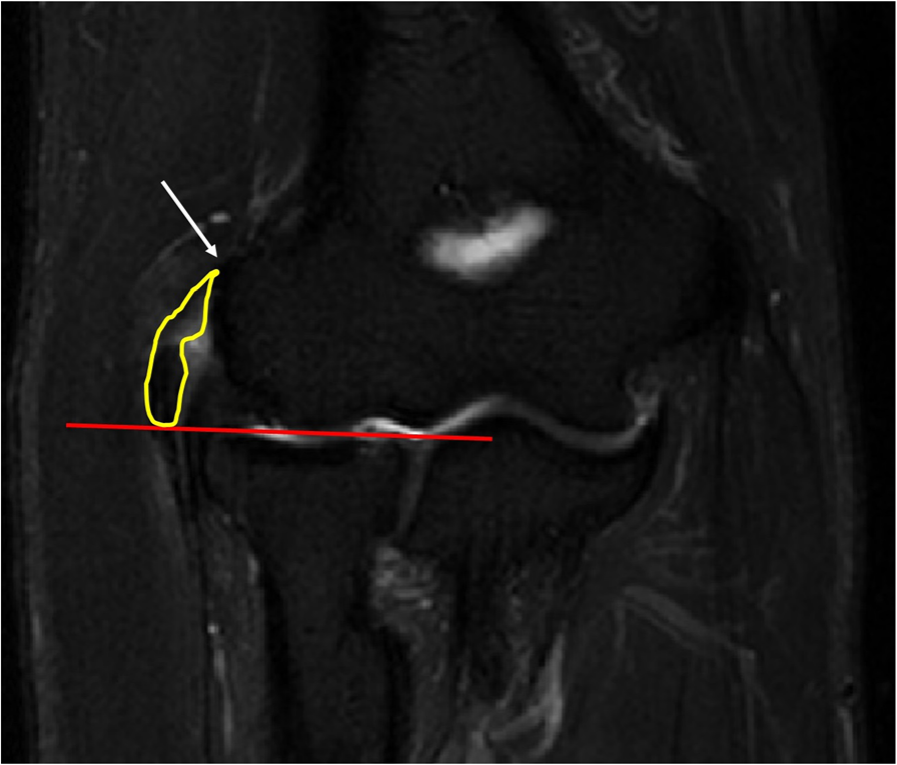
The proximal and distal boundaries of the common extensor tendon on the coronal fat-suppressed T2-weighted magnetic resonance imaging. The white arrow indicates the insertion site of the common extensor tendon located in the lateral humeral epicondyle, and the red line indicates the articular surface level of the radial head. A yellow-colored closed curve shows the margin of the region of interest of the common extensor tendon
